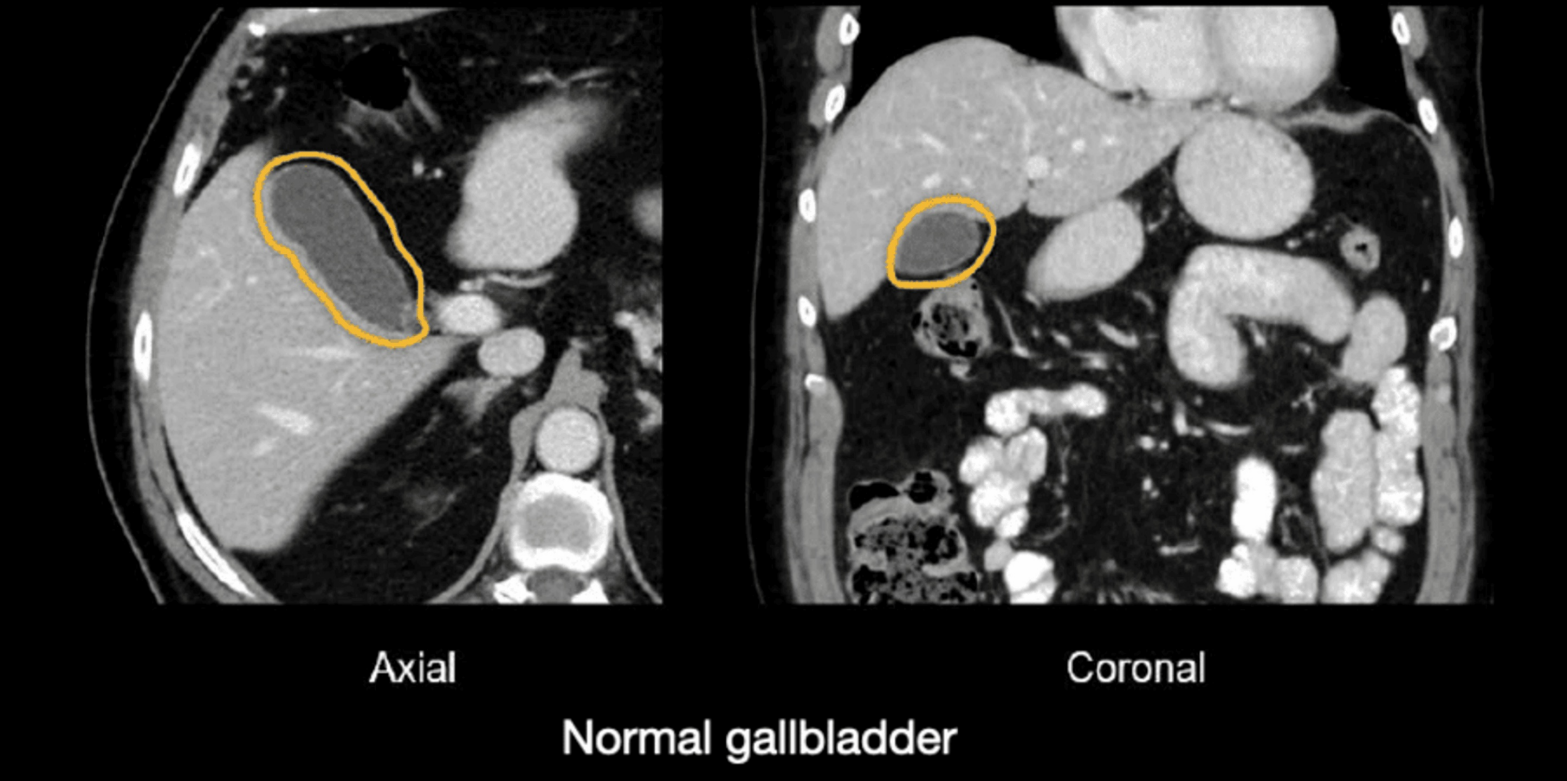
CT axial and coronal images showing normal gallbladder visualization. The image is reproduced from Hartung and Brown (2024) [4] under the CC BY 4.0 license.
